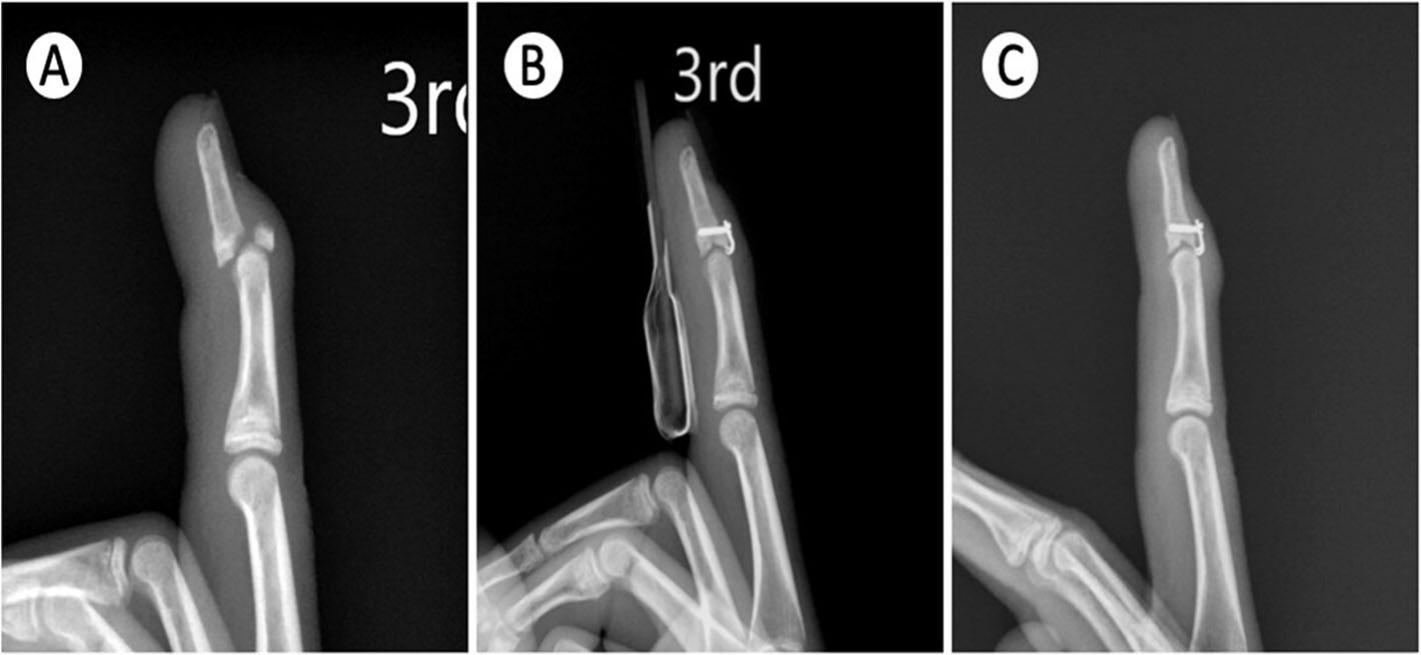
Union of mallet fracture. a The pre-operative radiograph showed mallet fracture with DIP joint subluxation. b The post-operative radiograph showed that the fracture was reduced and fixed with the hook plate. c Bone healing was shown on the 3-month follow-up radiograph
